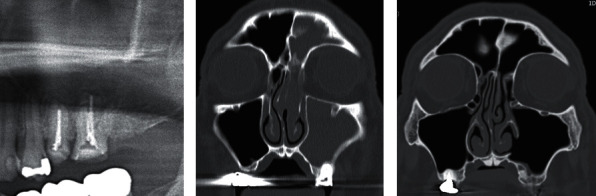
Radiographic findings. (a) Panoramic radiograph taken after the endodontic treatment of teeth #25 and #26 showing complete obturation of the roots except for the buccal distal root canal of #26. (b) Frontal plane of the preoperative computerized tomography (CT) scan revealed opacity extending from the left maxillary sinus to the left frontal sinus. (c) Frontal plane of the postoperative CT scan showed patency of the nasoantral window and the clearness of the left maxillary sinus and the left frontal sinus two years postoperatively.
